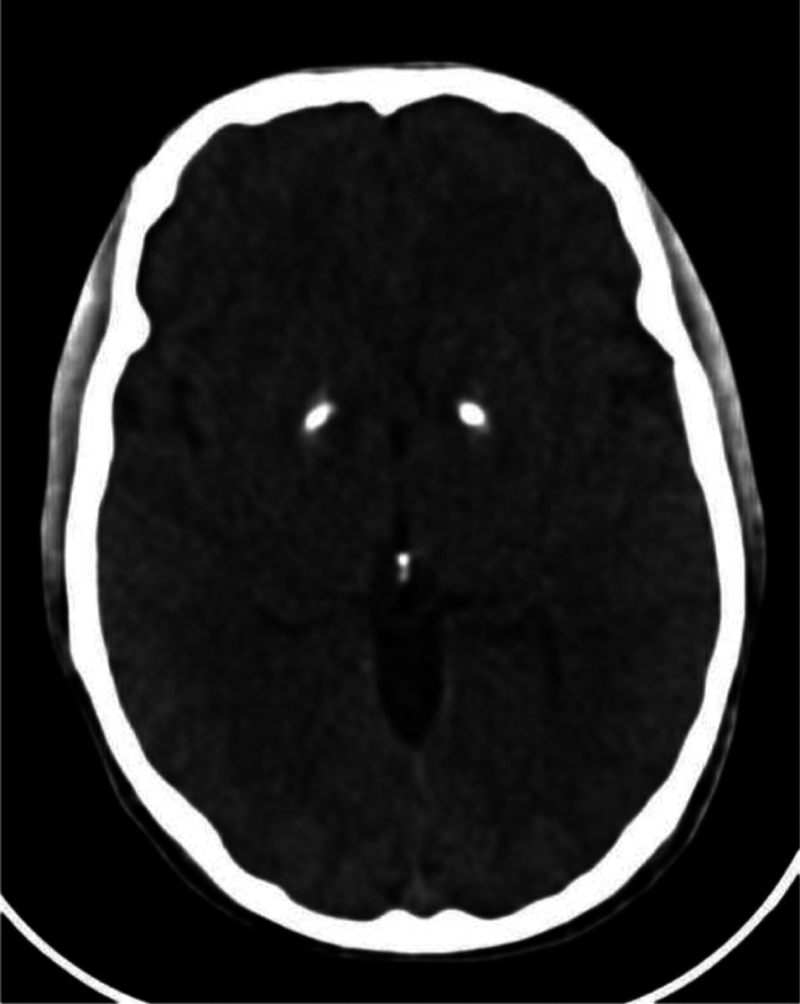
Skull computed tomography (CT) scan (performed in 2016): small and symmetrical calcifications on the basal ganglia.
